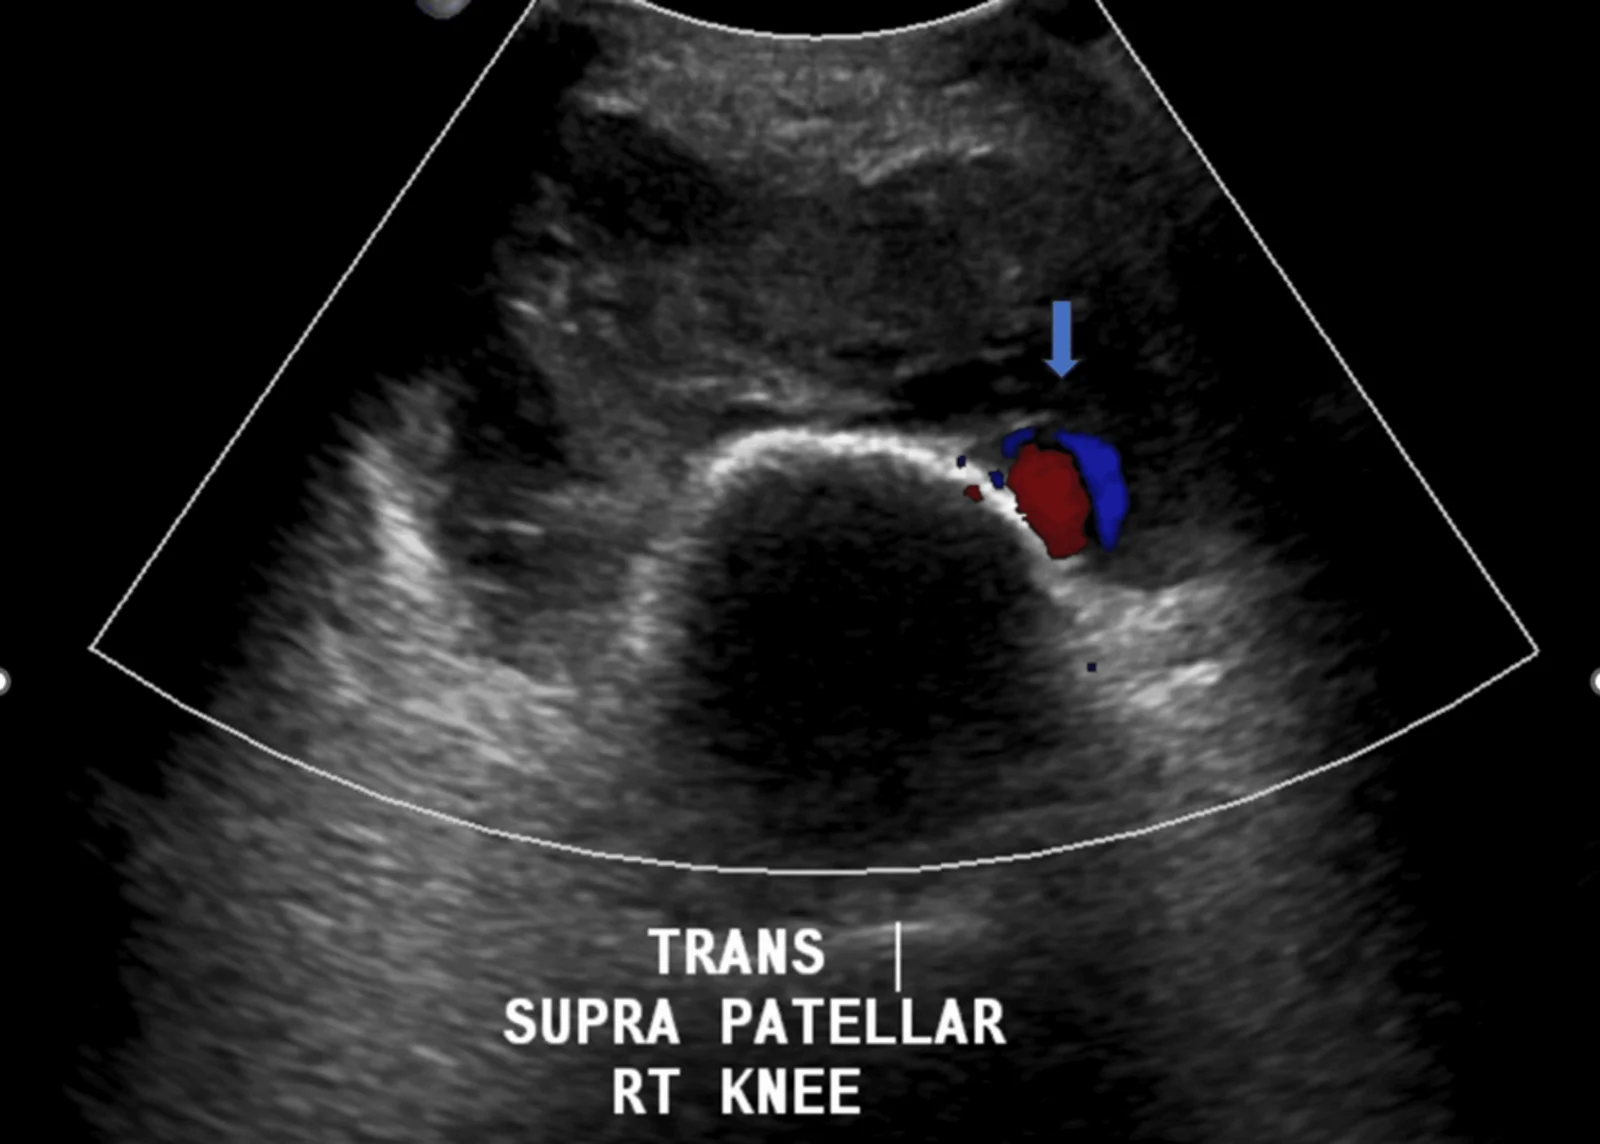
Ultrasound image of the knee Transverse ultrasound image of the knee demonstrating moderate iso- to hypoechoic complex suprapatellar joint effusion with synovitis. The blue arrow represents a focal yin-yang appearance on color Doppler images concerning for a small vascular lesion.
